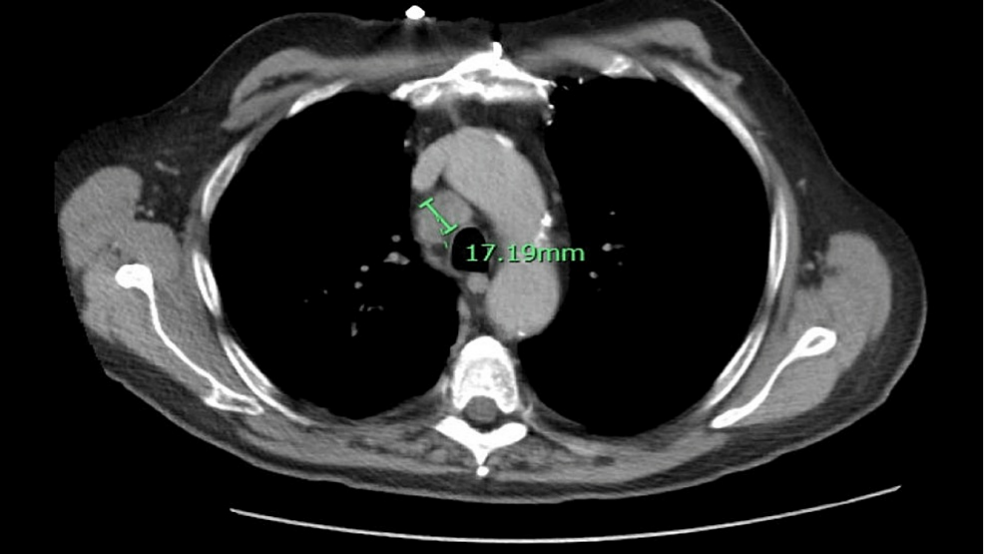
CT angiography of the abdomen, pelvis and pancreatic mass protocol showed a 1.7 cm pretracheal lymph node.
